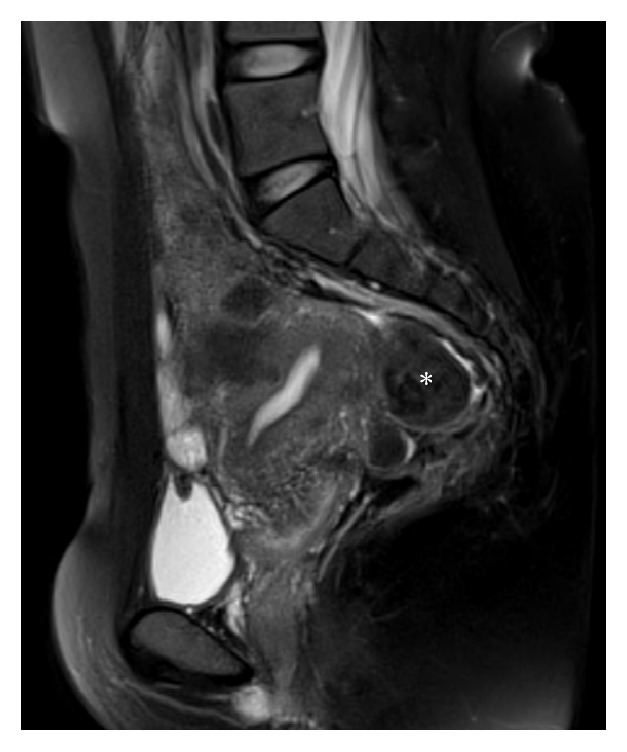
Sagittal T2-weighted MRI after delivery shows a large fibroid (white asterisk) located in fundus.
